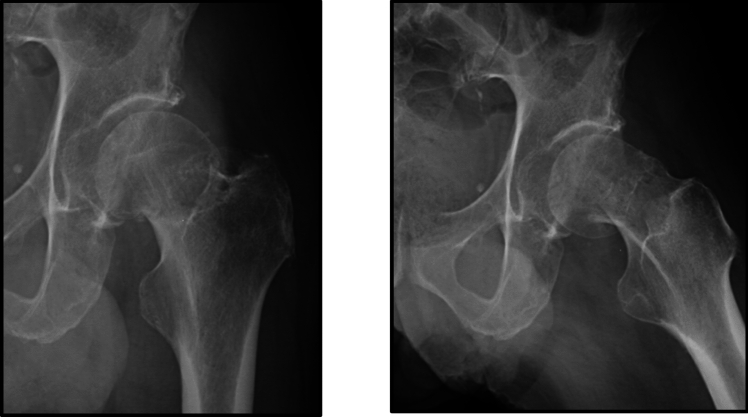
Radiographs of a posterior tilt between 15º and 20º
